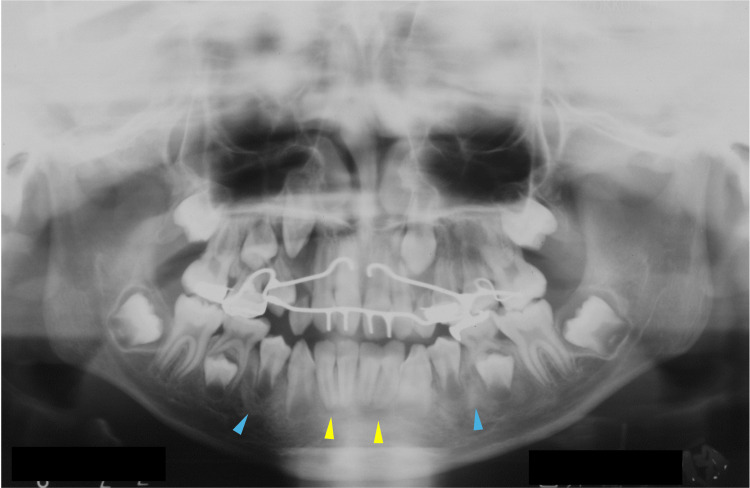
Maxillofacial radiograph at age nine Blue arrowheads indicate slight hardening of the bone lesions adjacent to the roots of the permanent mandibular teeth. Yellow arrowheads indicate a radiolucent area at the apical site of the lower frontal teeth
